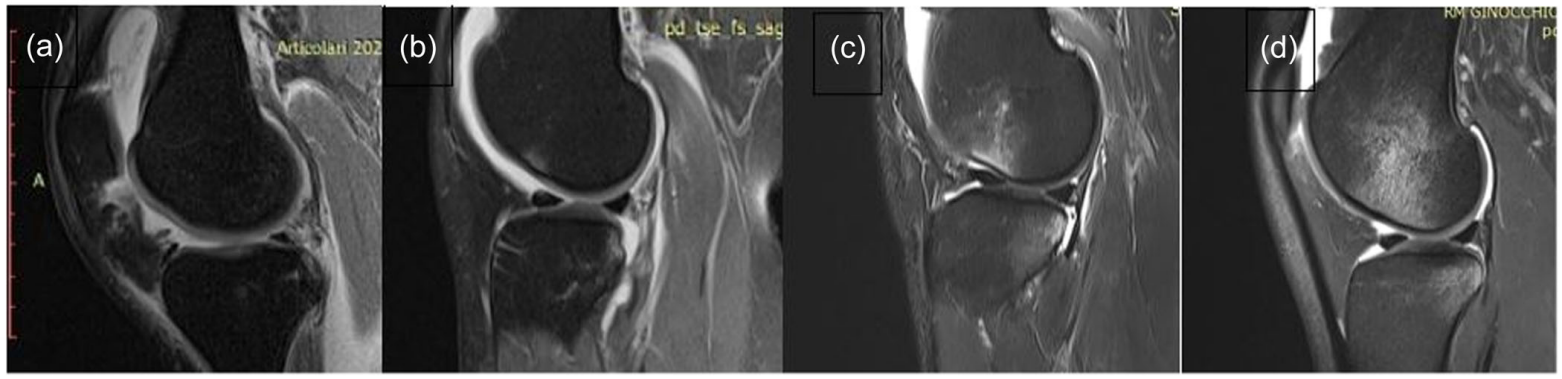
Classification of bone bruise into four grades for medial femoral condyle on magnetic resonance imaging according to the ICRS scale: a, absent; b, minimal; c, moderate; and d, severe [8]. ICRS, International Cartilage Repair Society.
